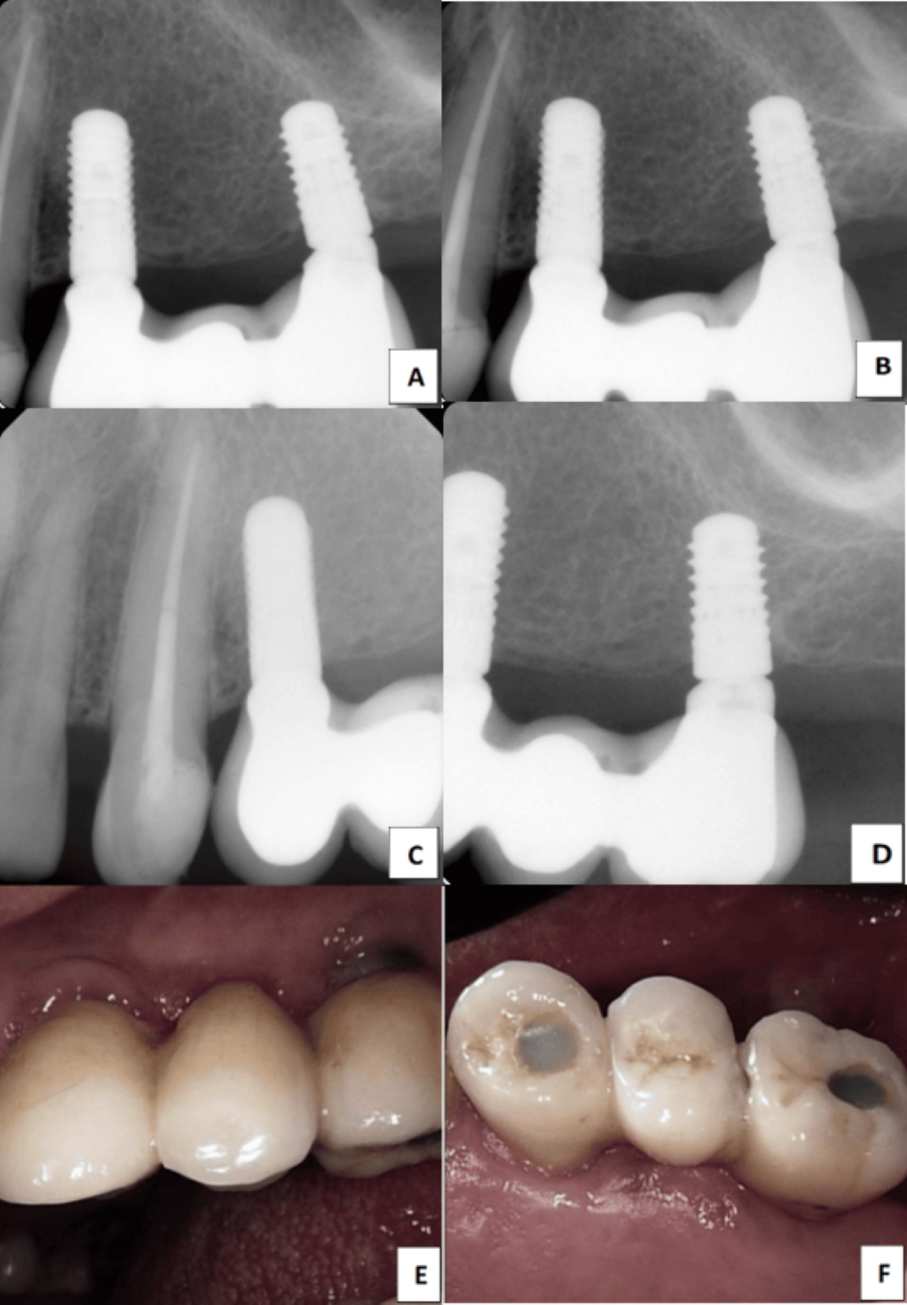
Follow-up images (A) The 2021 X-ray shows the two implants in the areas of #24 and #26. (B) The 2023 X-ray of the same implants. (C, D) The 2024 X-rays were taken for the #24 and #26 implants and prostheses. (E, F) Clinical photographs taken with an intra-oral camera in 2024, showing the same prosthesis after six years (E: Buccal view, F: Occlusal view).
